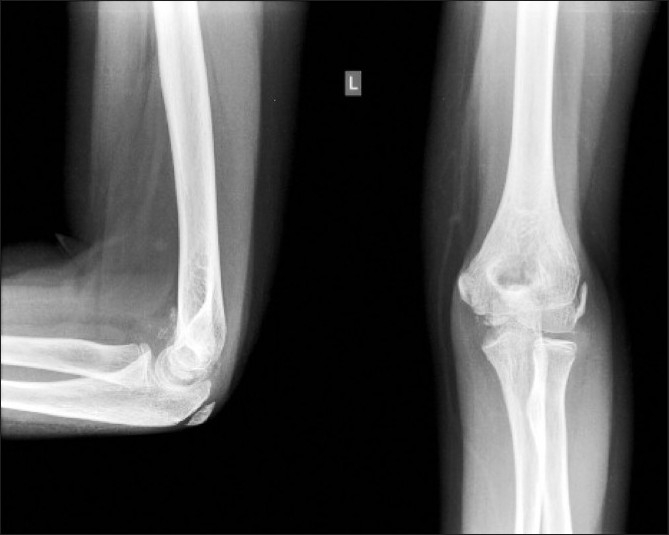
X-rays at three month follow up showing bone formation anterior and posterior to the distal humerus, suggesting a missed supracondylar fracture, capsular calcification and or loose bodies
